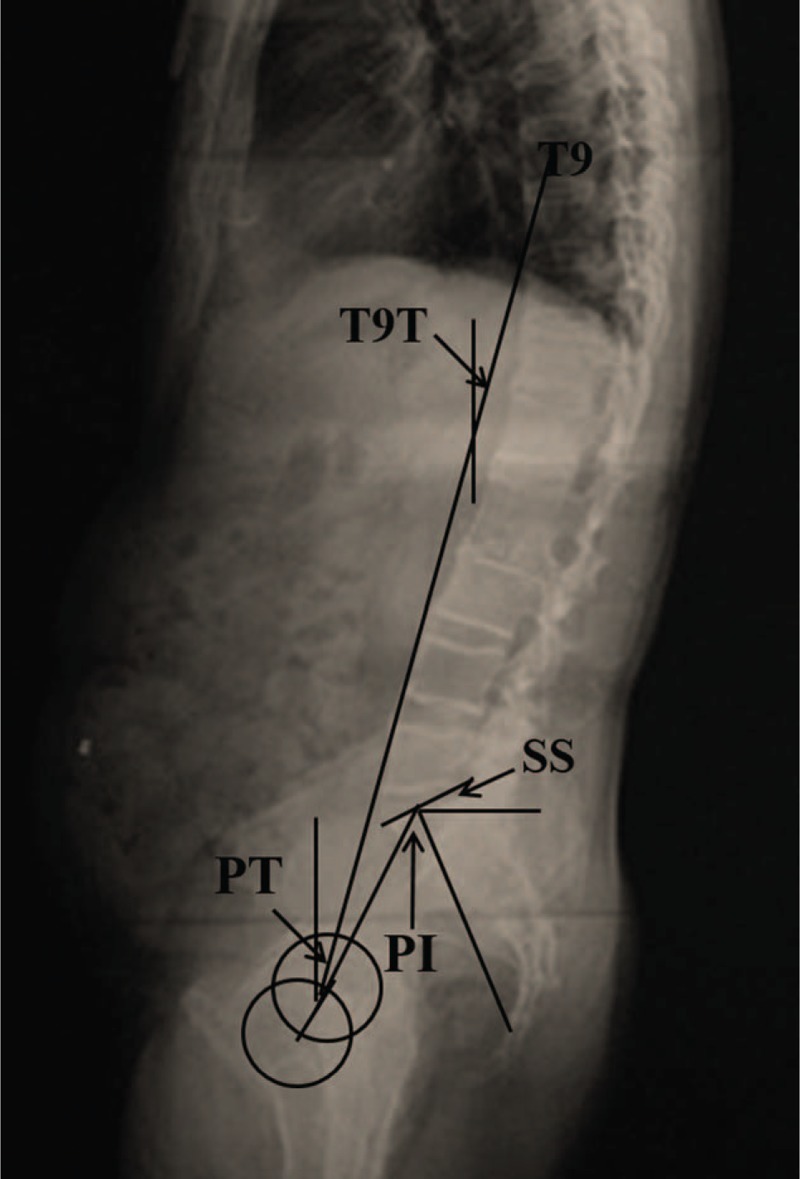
Sagittal pelvic parameters were measured from the standing lateral radiograph. PI is the angle between the line perpendicular to the sacral plate at its midpoint and the line connecting this point to the axis of the femoral heads. PT is the angle between the line connecting the midpoint of the sacral plate to the femoral head axis and the vertical axis. SS is the angle between the superior plate of S1 and a horizontal line. T9T is the angle between the vertical axis passing through the middle of both femoral heads’ centers and an axe passing through the center of T9 vertebral body. PI = pelvic incidence, PT = pelvic tilt, SS = sacrum slope, T9T = T9 tilt.
